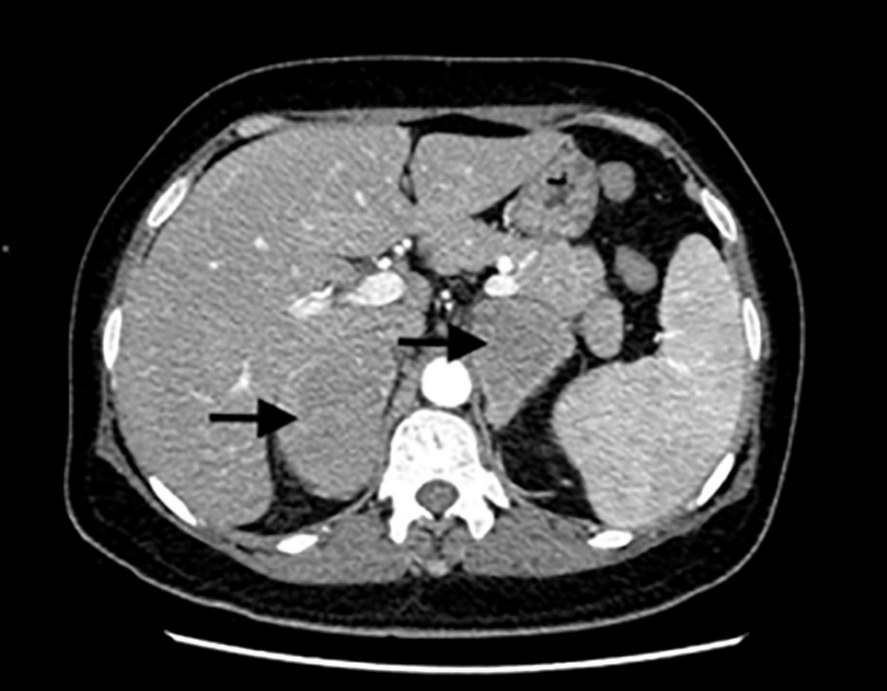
Abdominal CT scans indicated bilateral adrenal mass (noted by two black arrows). Enhanced CT showed bilateral adrenal mass with mild cord-like enhancement and slightly enlarged spleen.
